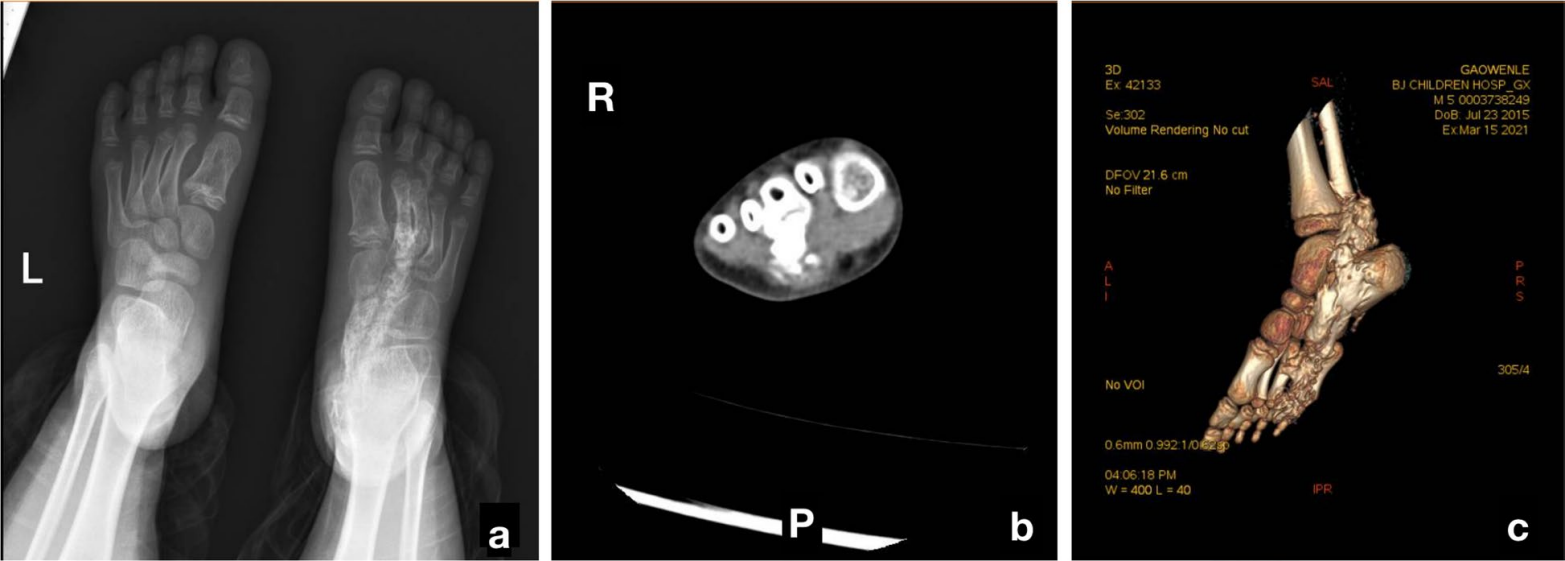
The radiographic image of the foot. AP right foot radiograph shows massive ossification centered along the third metatarsal from the toes to the hind foot. Some of the ossified areas have fused together (a). Axial CT of the patient shows ossification on the plantar aspect of the right foot extending between the second and third metatarsals with incorporation of the ossification in the third metatarsal (b). The tridimensional CT scan of the lower extremities reveled the range of the right foot calcifications (c)
